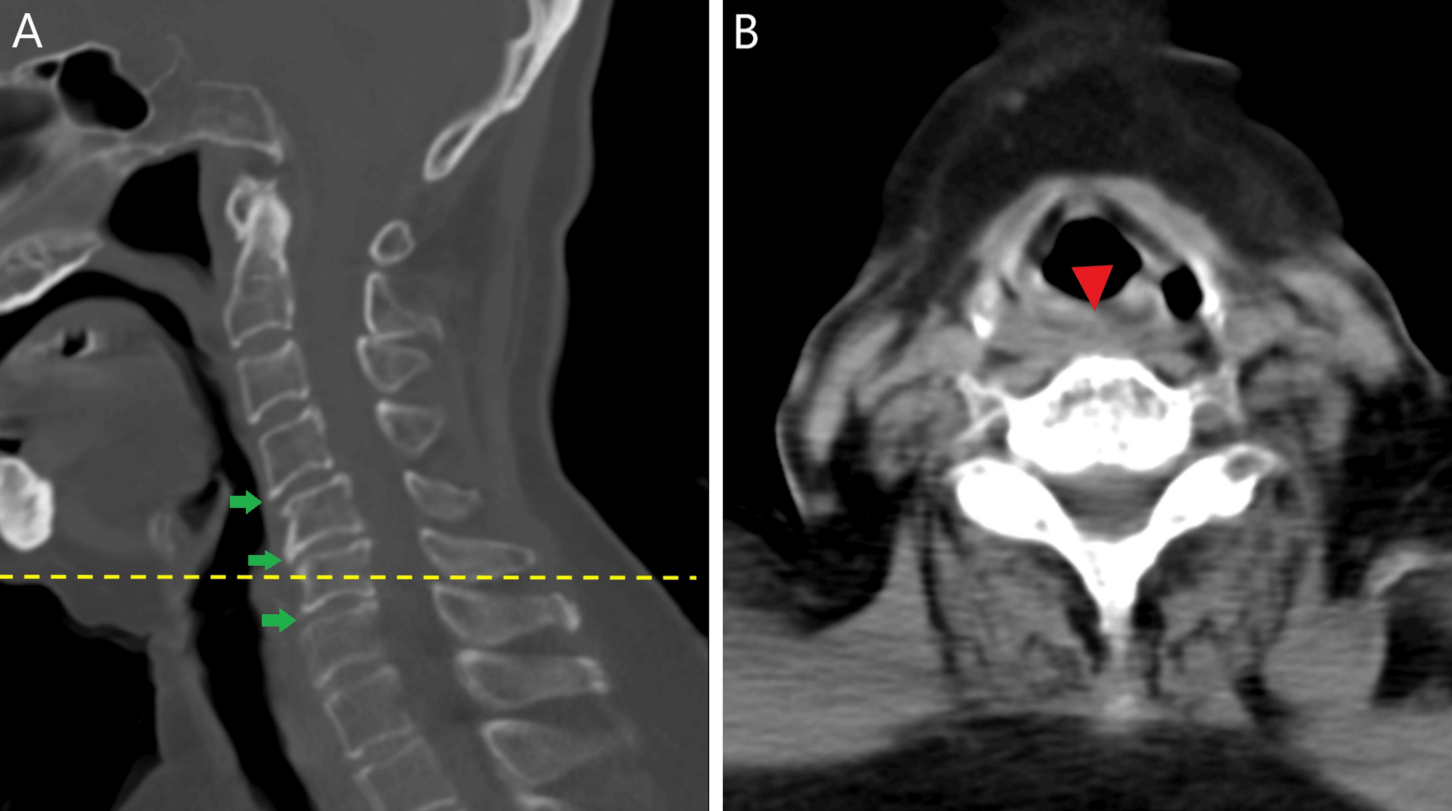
Cervical CT Cervical CT sagittal view (A) demonstrates mild spondylosis, disc space narrowing, and some spurs at levels C4–C7 (green arrow). The axial image (B) at C6 level (yellow dotted line) shows soft tissue swelling (red arrowhead), with no hypopharyngeal lesion and no evidence of cervical vertebral fracture no cartilaginous damage.
